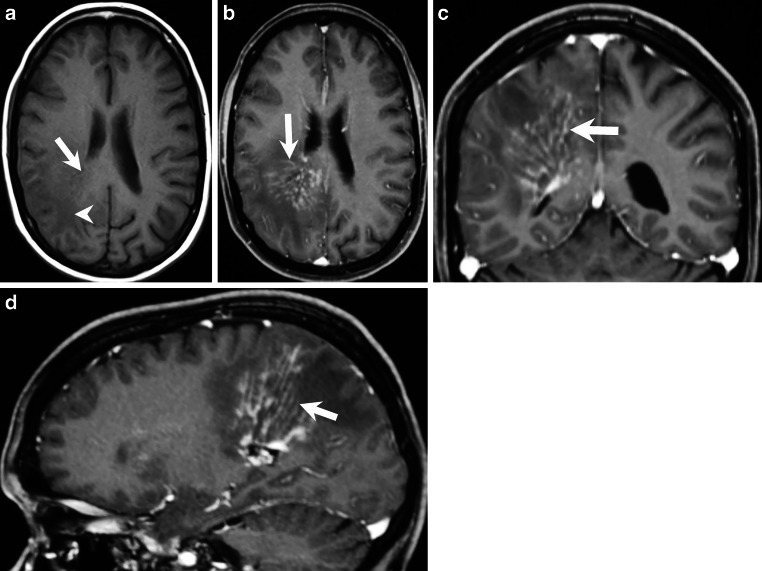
On axial, native T1-weighted images (a) the linear components of the lesion appeared hypointense (*arrow*) when compared to the cerebellar tissue with a distinct perifocal edema surrounding these structures (*arrowhead*). On axial (b), coronal (c), and sagittal (d) T1-weighted images after administration of gadolinium the linear structures show marked enhancement of contrast (b–d, *arrowhead*) most likely corresponding to perivascular spaces
